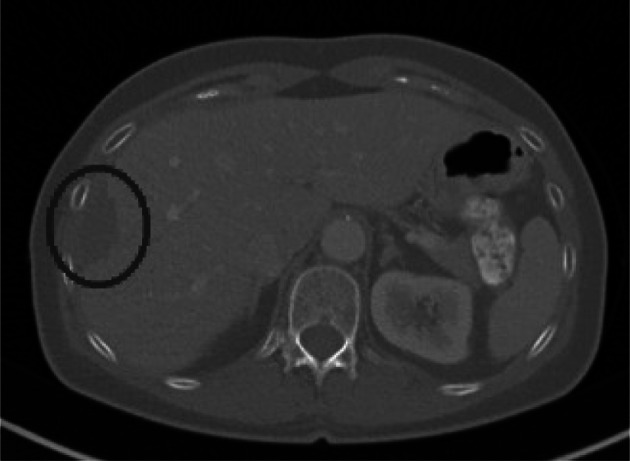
Part of original CT image with marked tumor
